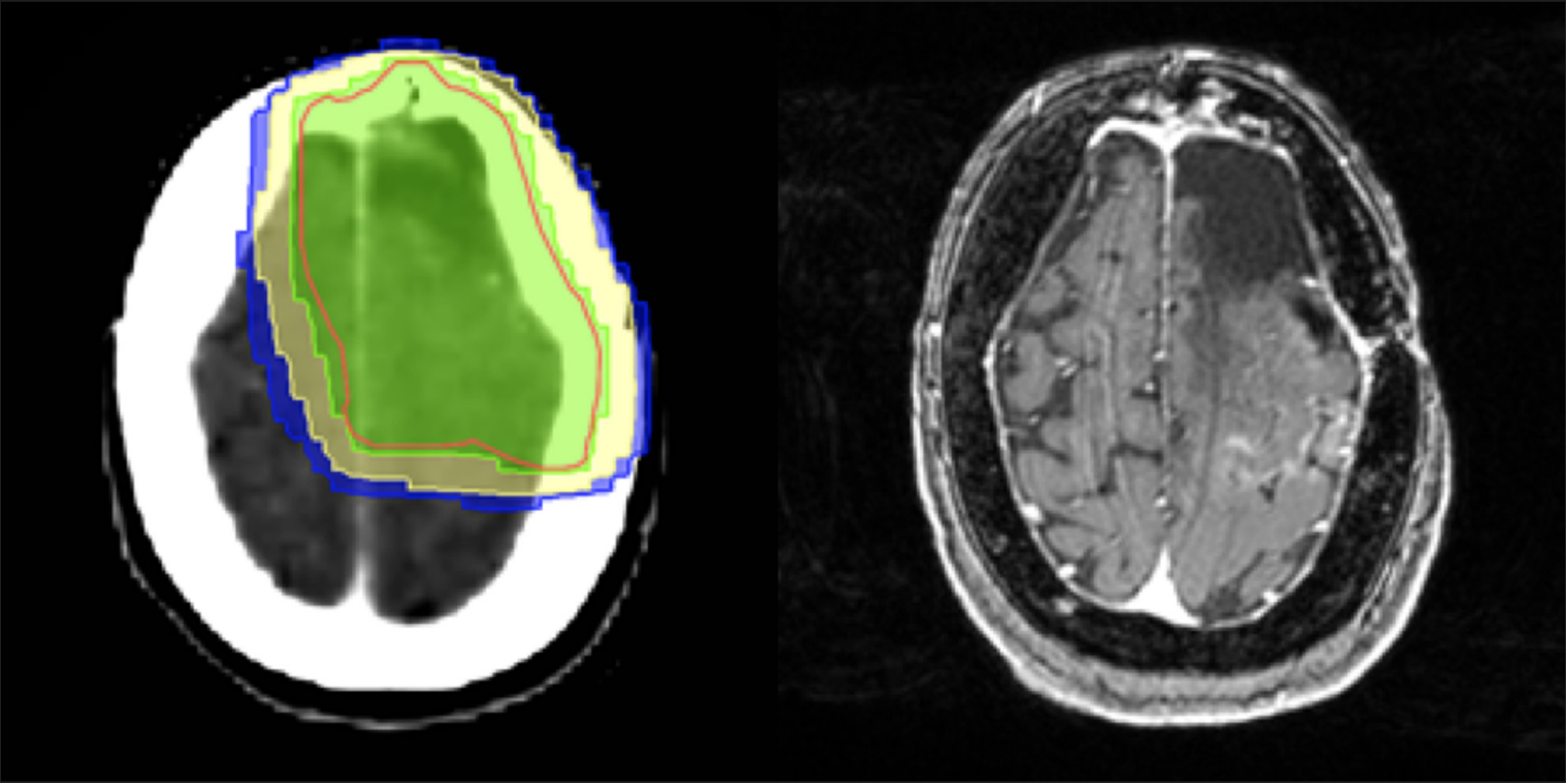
Radiotherapy dose distribution with correlating axial post-contrast T1-weighted image demonstrating the cortical enhancement at presentation.
